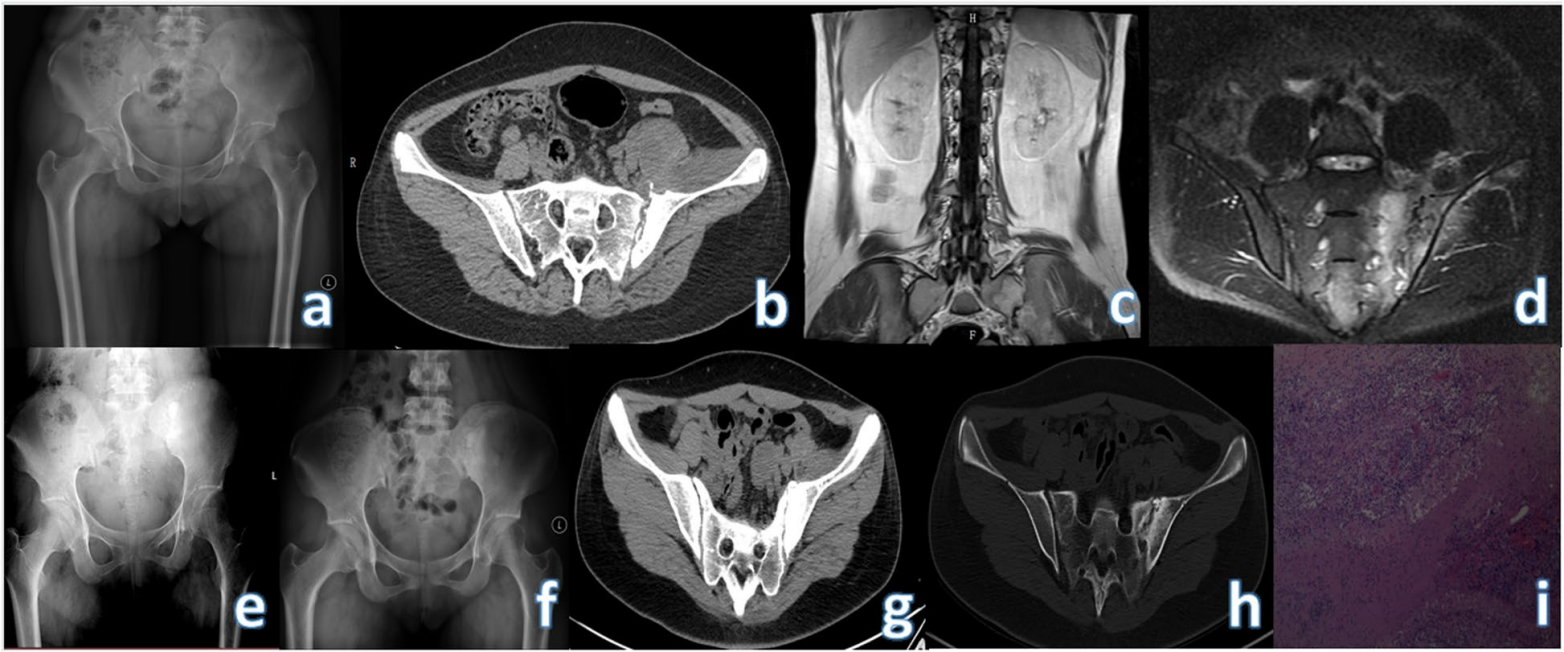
Preoperative X-ray, CT and MRI of the patient, as well as X-ray at 3 months, 6 months after operation and CT at 12 months after operation of NO.4. a-d Preoperative X-rays, CT and MRI: the right sacroiliac joint space is indistinct and part of the bone is destroyed; the left iliac muscle and the front of the hip joint can be seen with irregular and mixed density soft tissue shadows; the psoas major muscle can be seen with patchy slightly Long T2 signal. e X-ray at 3 months after operation: it can be seen that the bone graft is not fused with the joint. f X-ray at 6 months after operation shows the fusion of the bone graft and the joint. g-h There is no bone resorption in the bone graft part, and the joint part is fused in the CT at 12 months after operation
